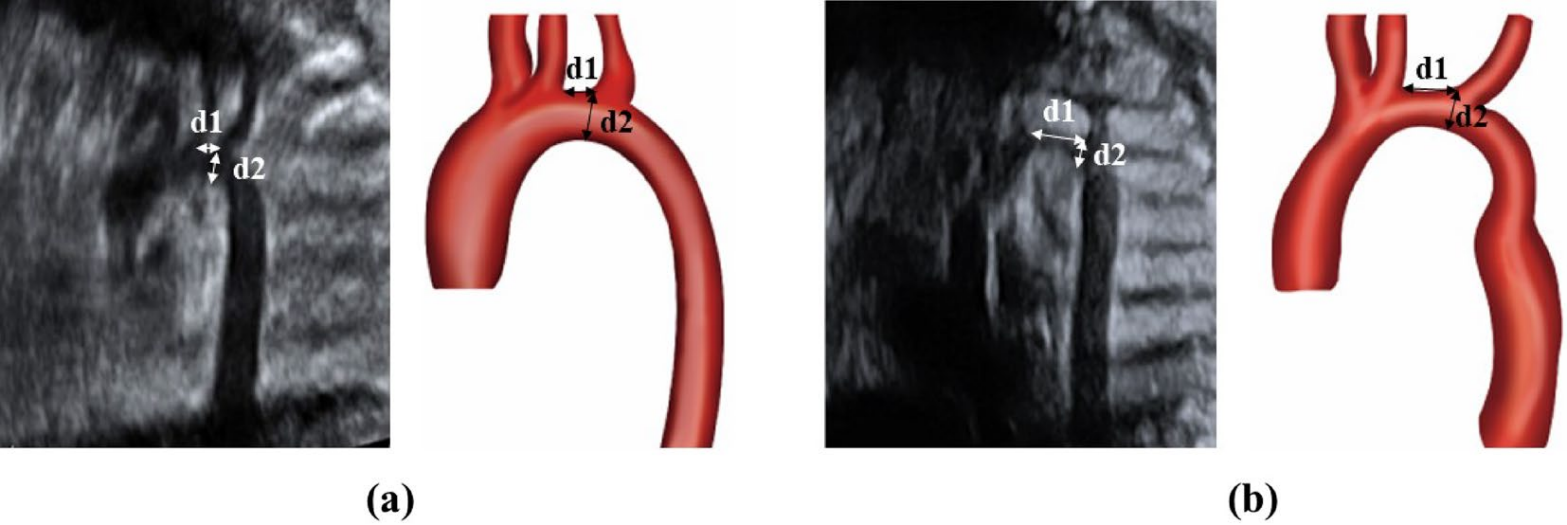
Echocardiographic and enhanced computed tomography scheme of an aortic arch (DA index = d1/d2). Echocardiographic and enhanced computed tomography image of a normal aortic arch with normal DA index (a) and CoA with abnormal DA index (b). The DA index was the ratio of the distance between the origin of the left carotid artery and the origin of the left subclavian artery (d1) to the diameter of the distal aortic arch at the origin of the left subclavian artery (d2). *DA* distal aortic arch, *CoA* coarctation of the aorta.
